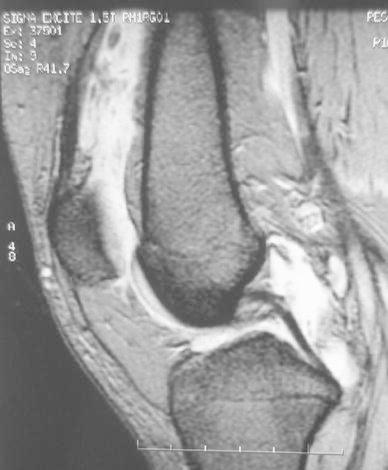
Sagittal reconstruction of the MRI showing villous-like projections invading the suprapatellar pouch with a fat signal intensity on T2
